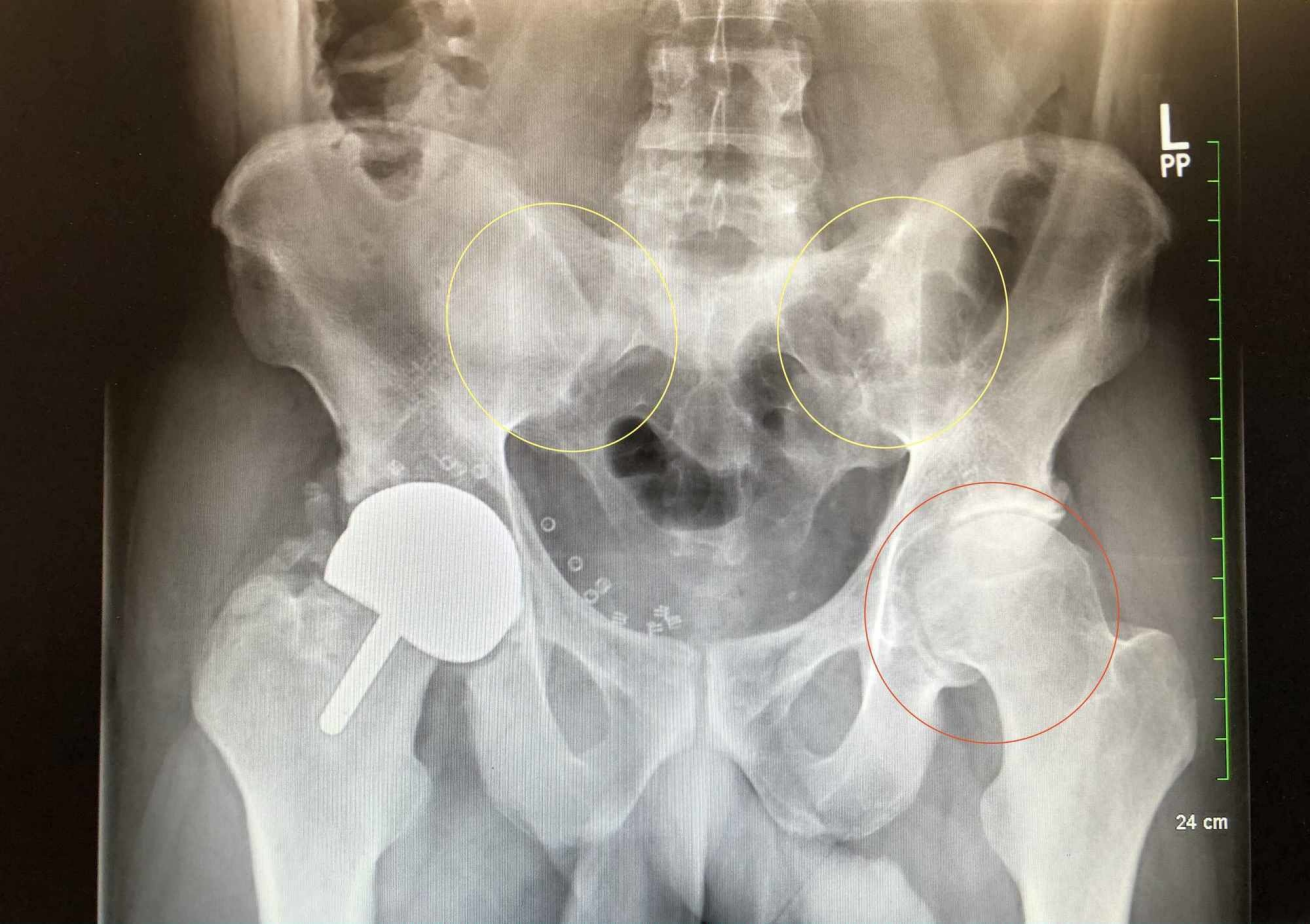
X-ray Image of the sacroiliac spine Findings: There appears to be a fusion of the sacroiliac (SI) joints (yellow circles). There is a marked narrowing of the left hip joint (red circle). There is a hip resurfacing prosthesis on the right. Impression: There is a fusion of the SI joints. Prominent degenerative change and narrowing of the left hip joint. Resurfacing prosthesis on the right hip.
